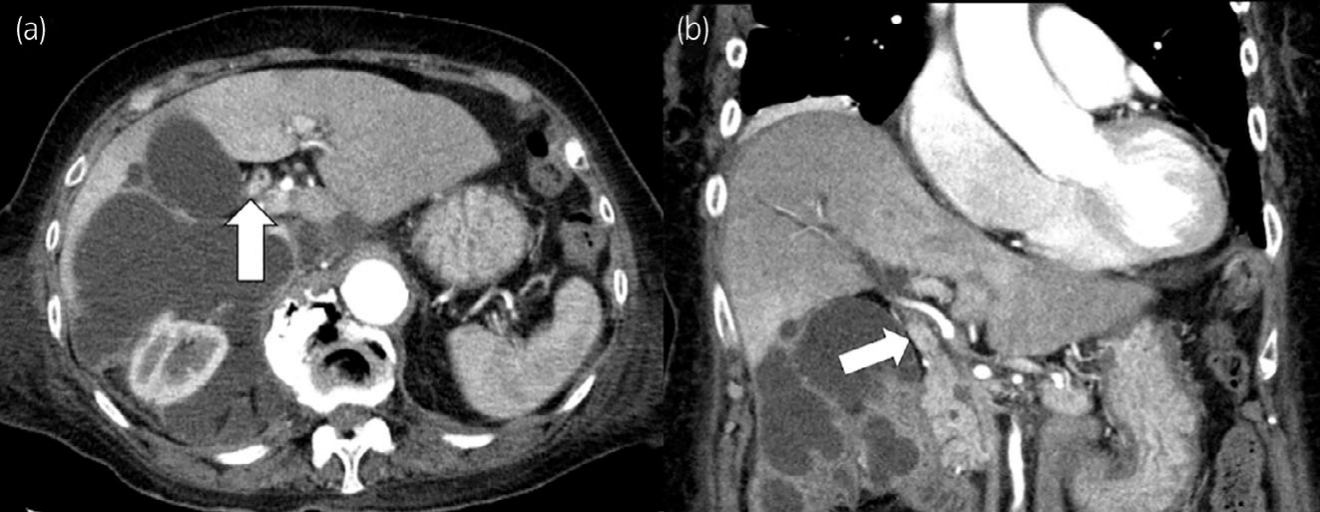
Contrast‐enhanced CT of the abdomen showed an increase in accumulation of multifocal fluid with septal contrast effect. Soft tissue in the common bile duct demonstrated contrast effects (arrows). (a) Axial section, (b) coronal section.
